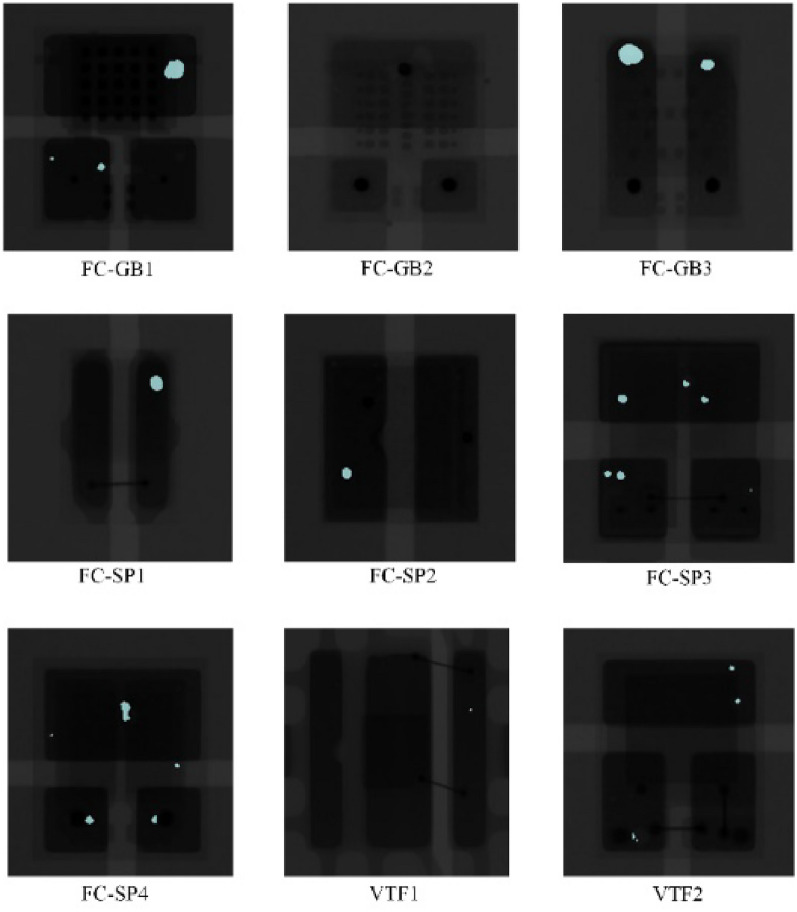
X-ray images of 9 kinds of LED chips in DeeplabV3 model test result display.
